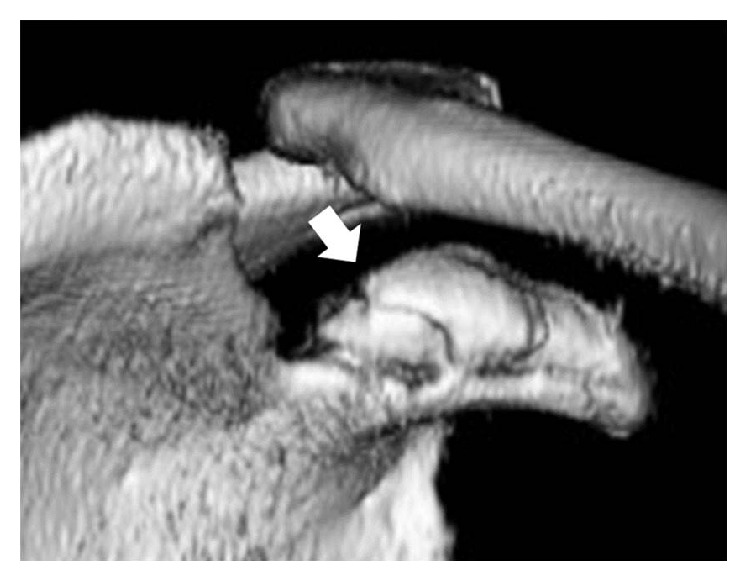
A 3D-CT reconstruction clearly reveals a small bone fragment at the CP medial apex (arrow).
